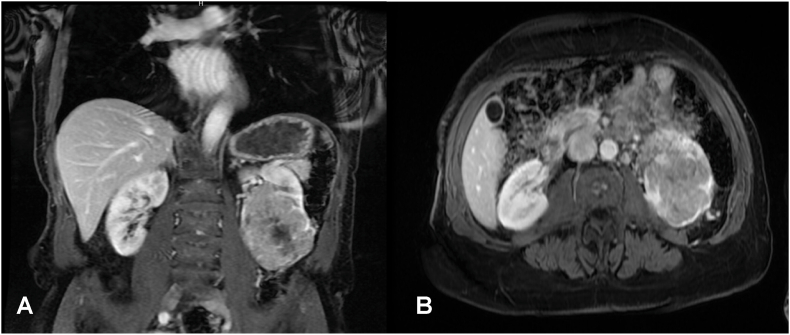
Computed tomography (CT) imaging A. CT abdomen/pelvis demonstrating an 8.8 × 6.3 cm exophytic mass arising from the lower pole of the unenhanced left kidney - coronal view B. Left renal mass with subcentimeter retroperitoneal lymphadenopathy - axial view.
